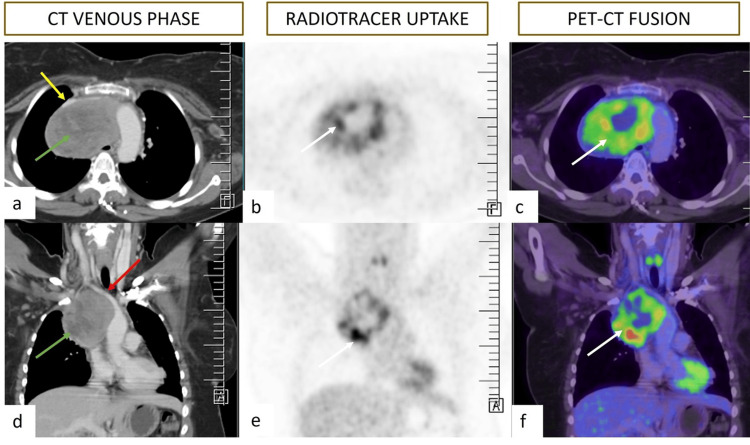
Axial (a, b, and c) and coronal (d, e, and f) reformatted PET-CT images show an FDG-avid heterogeneous mass lesion (SUVmax: 6.7) in the superior mediastinum, anterior and right to the trachea. Non-enhancing necrotic areas are seen within (green arrow). The superior vena cava is compressed and pushed anteriorly (yellow arrow). The right brachiocephalic trunk is pushed superiorly (red arrow). PET-CT: positron emission tomography-computed tomography, FDG: fluorodeoxyglucose, SUVmax: maximum standardized uptake value
